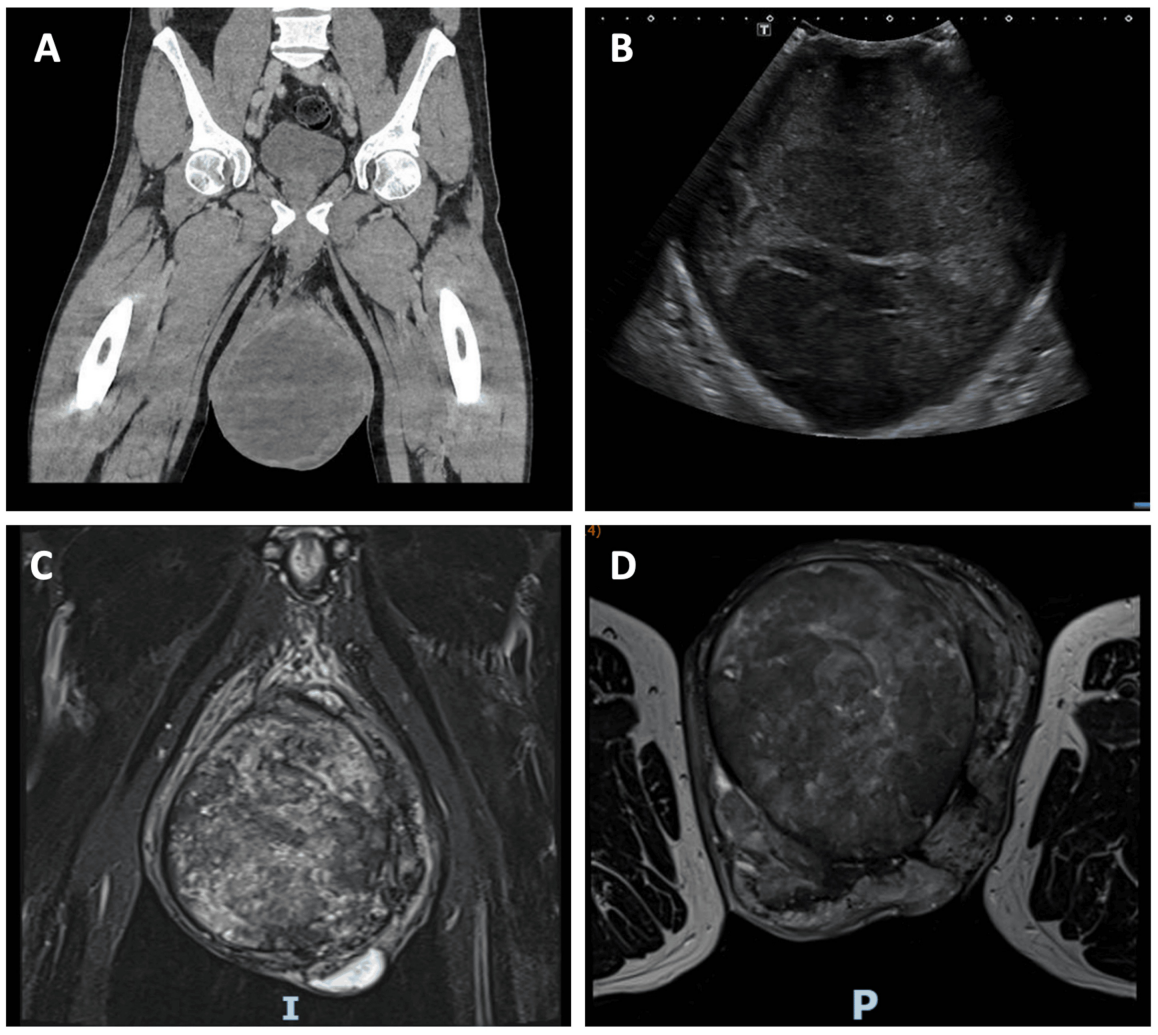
Summary of the three imaging modalities used A: coronal view contrast-enhanced CT; B: greyscale view ultrasound; C: coronal view T2-weighted MRI; D: axial view T1-weighted MRI
